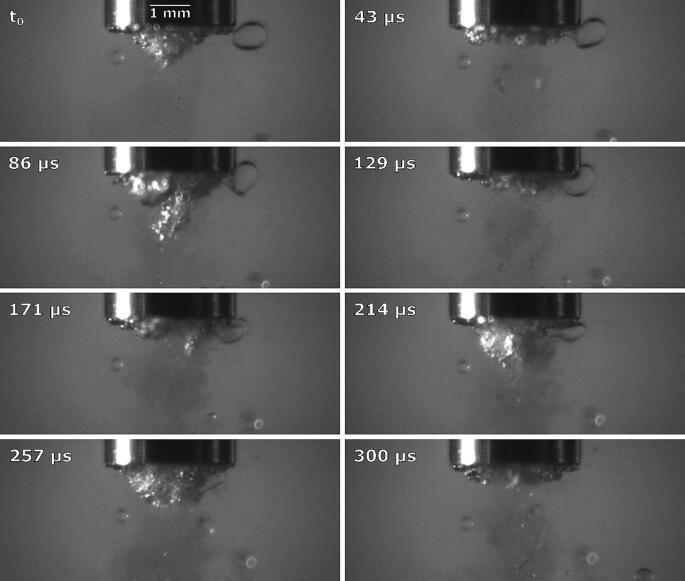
Photron SA-Z observations under visible light of a large oil droplet (near the tip’s right corner) collapsing into fine emulsion due to cavitation underneath the ultrasonic horn.
